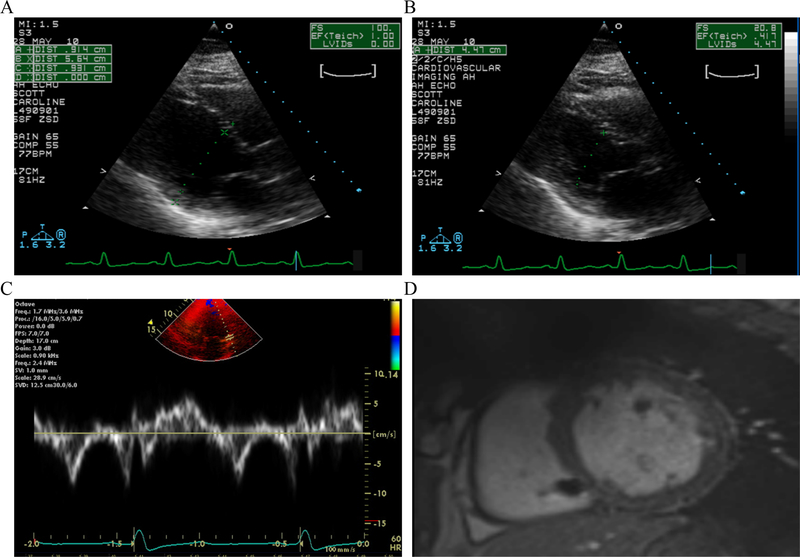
Echocardiogram and cardiac magnetic image resonance in the proband. Panels A and B show parasternal long axis view of the ventricles in diastole (panel A) and systole (Panel B). The left ventricular end diastolic and systolic diameters were measured 5.64 and 4.47 cm, respectively, and a calculated left ventricular fractional shortening of 21%. Panel C shows myocardial tissue Doppler velocities depicting low velocities. Panel D shows cross sectional myocardial images obtained by magnetic resonance imaging, which shows enlarged ventricles and absence of fibrofatty infiltration of the myocardium.
